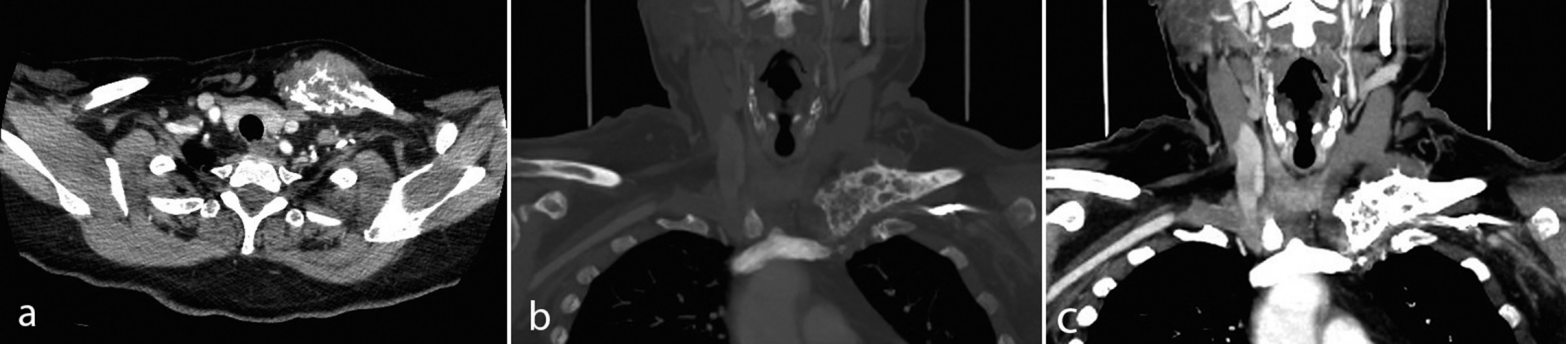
(a) Axial CT scan with i.v. contrast medium showing an expansile, predominantly lytic lesion of the medial left clavicle with associated tissue mass using soft tissue windows. (b) Coronal CT scan with i.v. contrast medium demonstrating the mass at the medial left clavicle using bone windows. (c) Coronal CT scan with i.v. contrast medium demonstrating the mass at the medial left clavicle using soft tissue windows.
